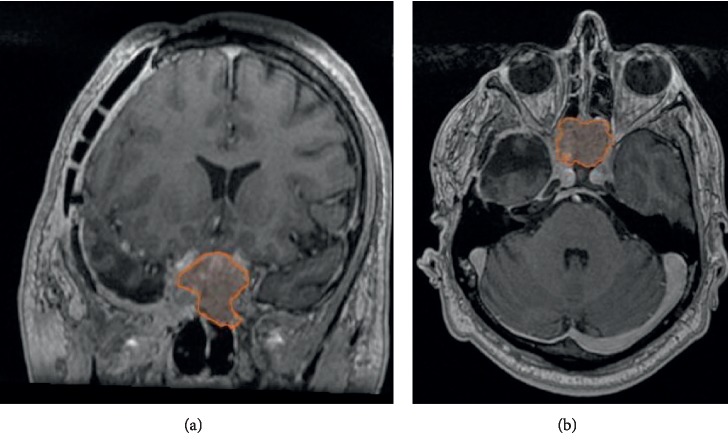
Follow-up MRI with residual tumor lesion near the sellar region extending to nasal cavity with 11.9 cm^3^ volume.
